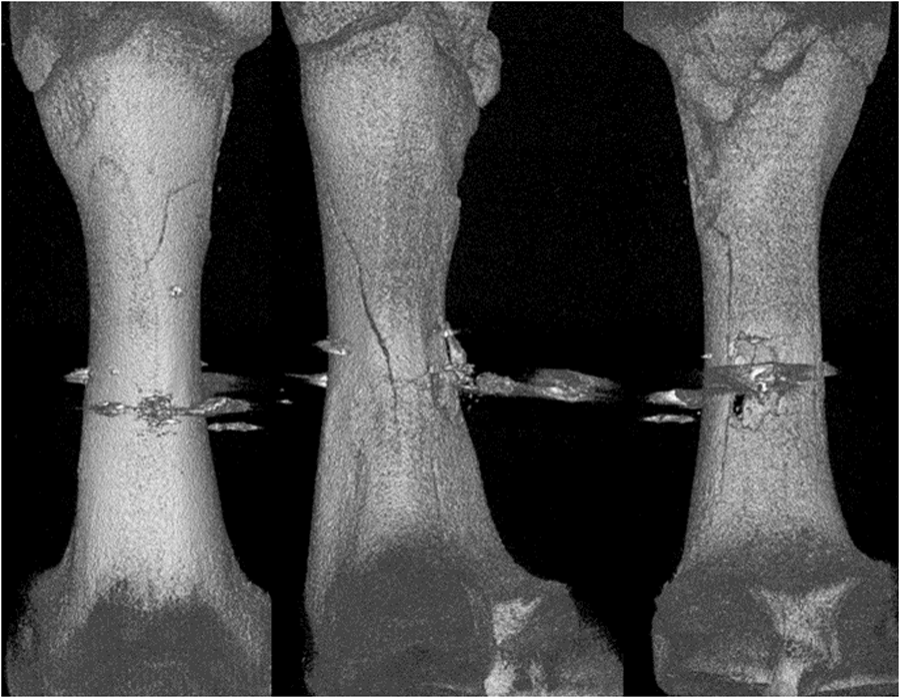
H&N Baracuda cal. 5.5 mm. 3D CT gunshot damage reconstruction. From the left: anterior, lateral and posterior shaft surfaces.
